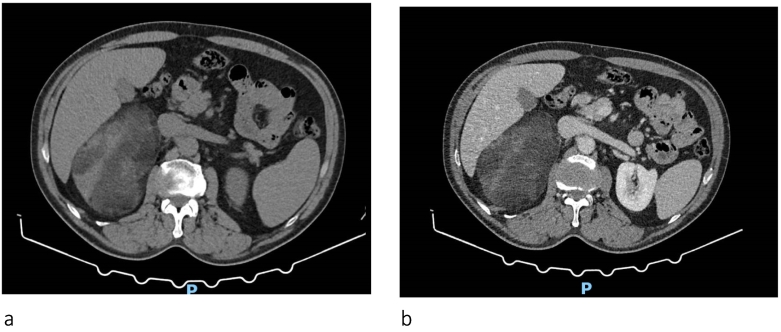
CT scan with basal acquisition (a) and with contrast (b). Voluminous, solid lesion with poor acquisition of contrast and well-defined, regular contours.
